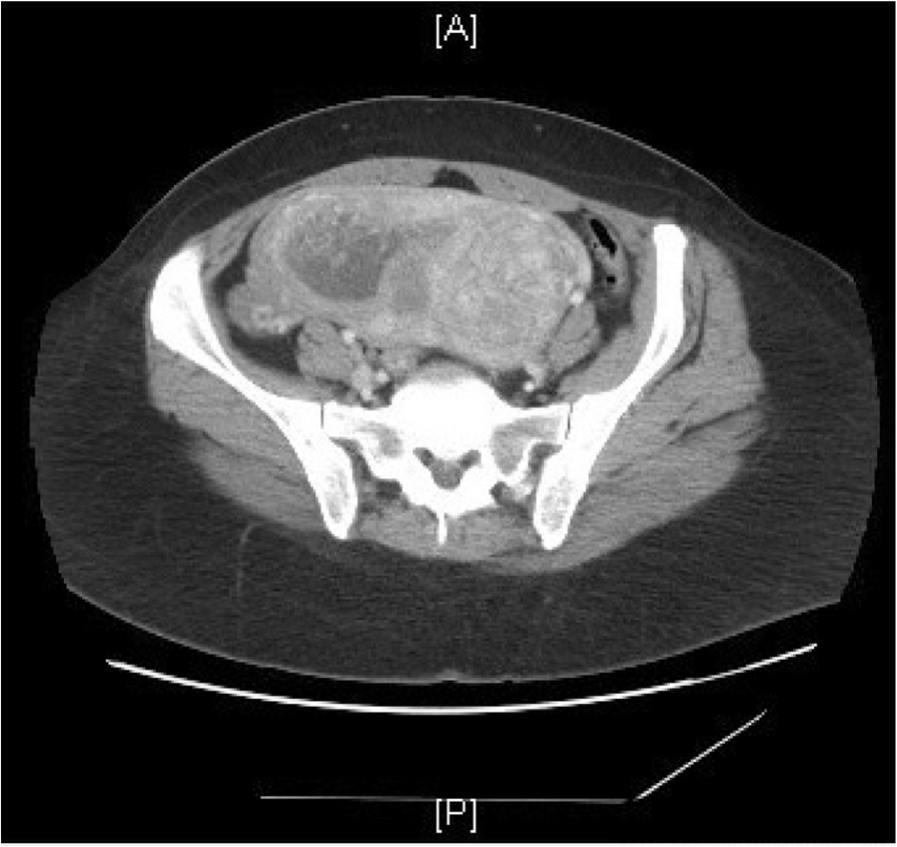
Axial view – Abdominal CT scan with an enlarged uterus due to a complete molar pregnancy
